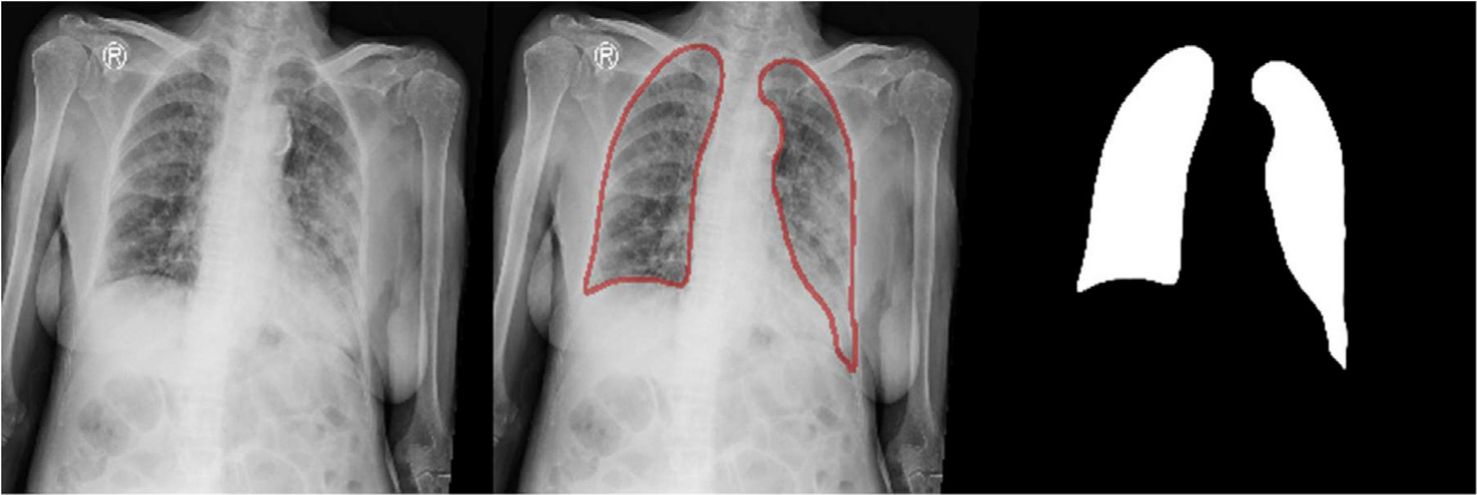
Merge of chest X-ray Image, Line annotation image, and segmentation image that shown to radiologist.
